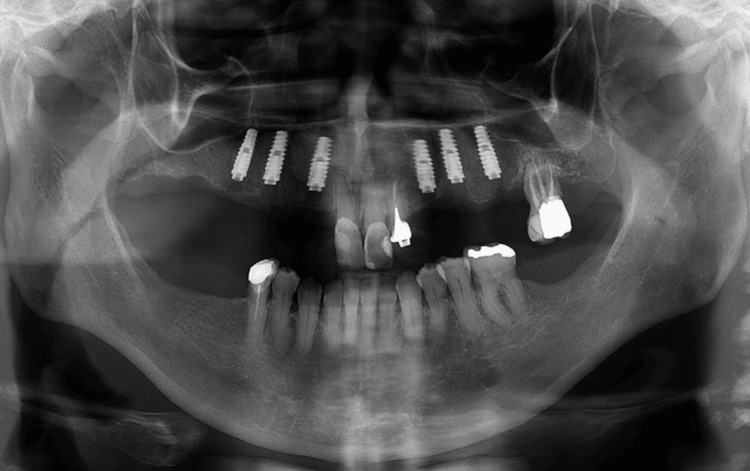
Panoramic radiograph seven days after implant placement
